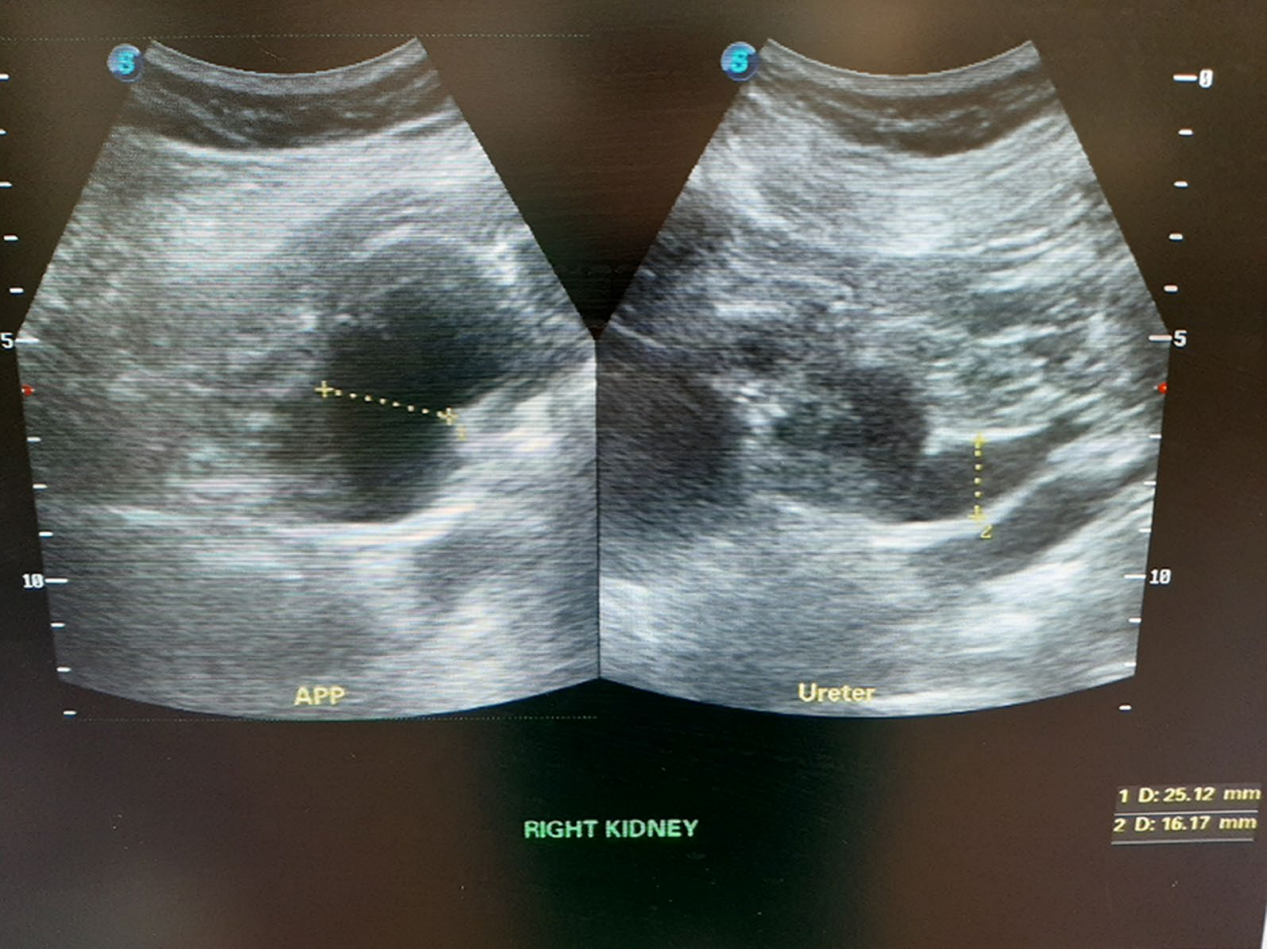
Ultrasound with right hydroureteronephrosis
